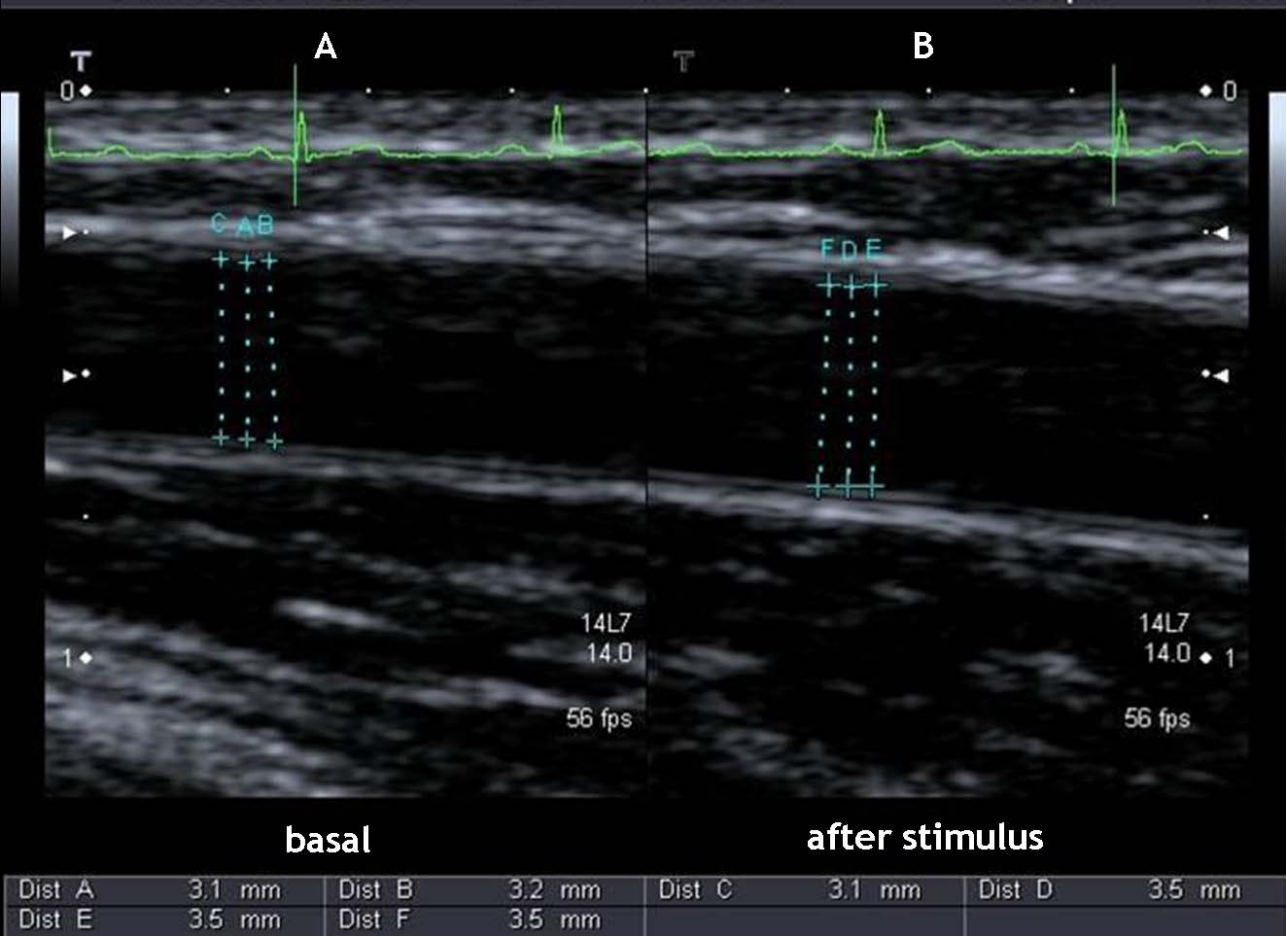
Ultrasound image of the brachial artery at (A) baseline and (B) 60 seconds after hyperaemic stimulus.
